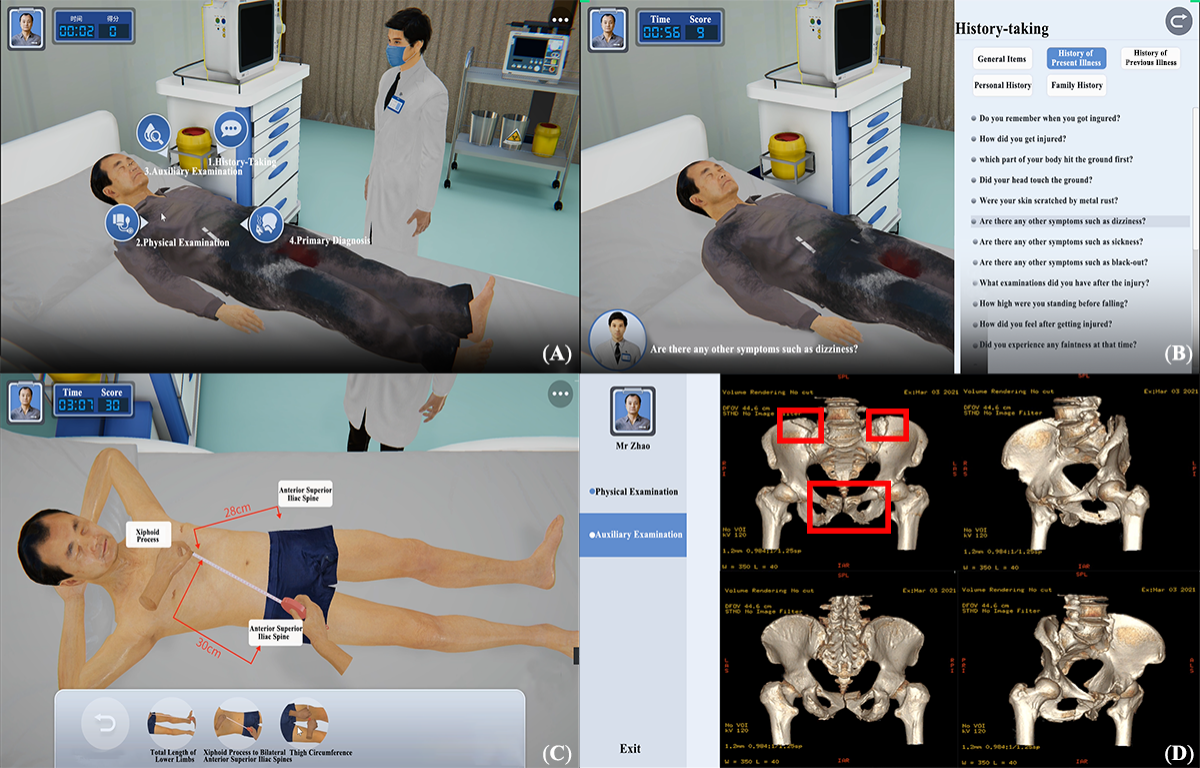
Screenshot of the emergency room. (A) The user acts as a doctor in the emergency room while interacting with the ESP by selecting different diagnoses and treatment icons. (B) Trainees can choose the present history module in history taking to communicate with the ESP. (C) During the physical examination session, students can use a tape measure to measure the distance between the bilateral anterior superior iliac spine and the xiphoid process to determine whether the pelvis was displaced. (D) The students order a computed tomography scan to evaluate the location and severity of the ESP’s injury. ESP: electronic standardized patient. Please note that a higher resolution version of this image can be found in Multimedia Appendix 1.
